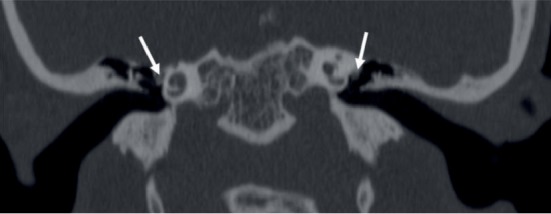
Coronal high resolution CT Temporal bone image showing bilateral dehiscent tympanic segments of facial nerve (arrows), abutting the lateral wall of the bony labyrinth due to its inferio-medial anomalous course.
